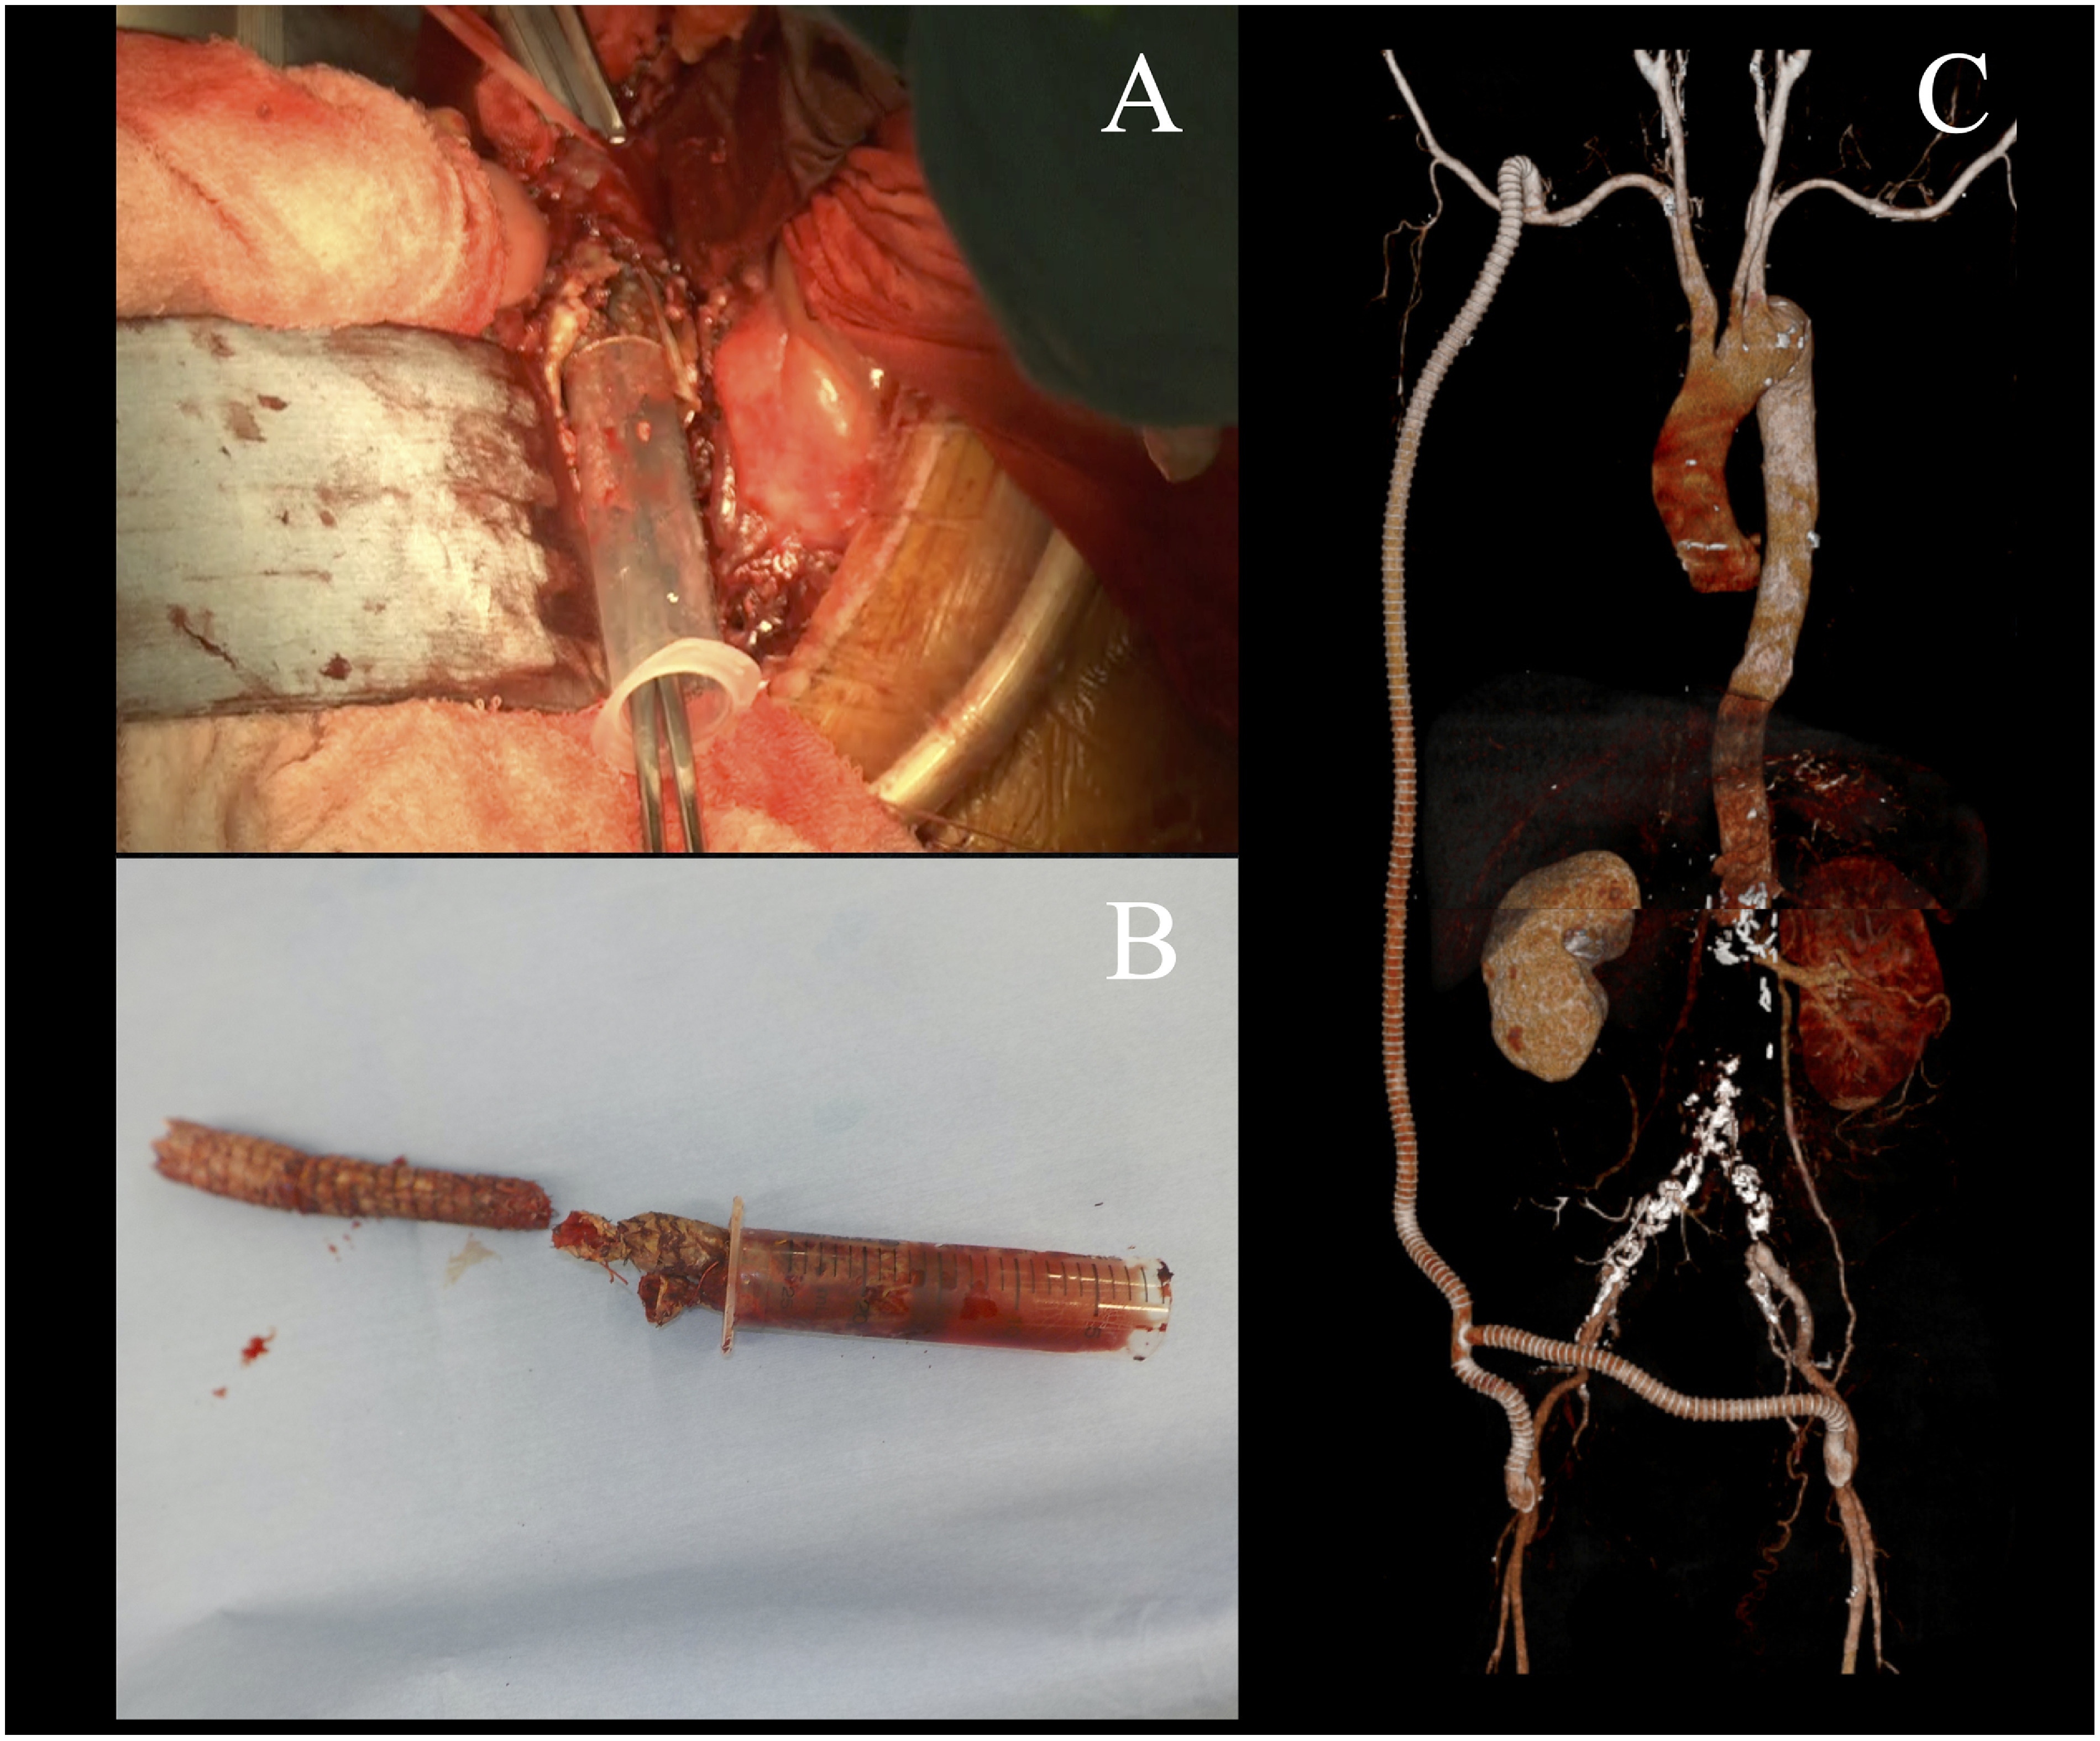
Fig. 3 (A) The infected stent-graft was removed using a 20-milliliter syringe. (B) Removed infected stent-graft. (C) A postoperative three-dimensional computed tomography showed the right axillary-bifemoral bypass.
